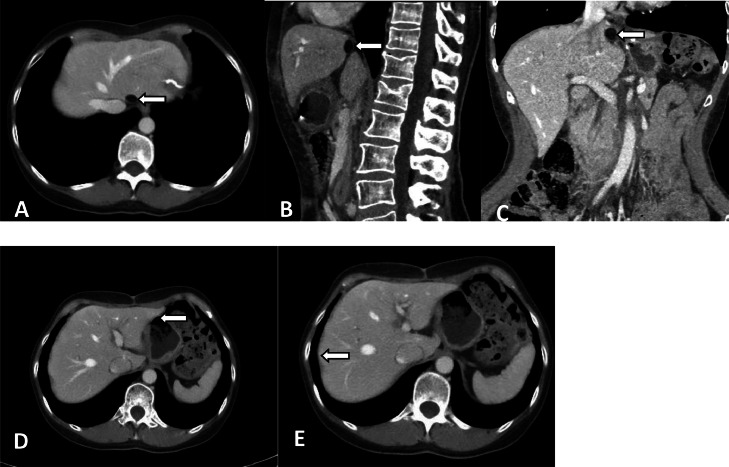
Reexamination using computed tomography (CT). On May 9, 2018, re-examination via CT showed a dense, fatty lesions (A-C) in the space between the left and caudal lobes of the liver under the hepatic capsule. The lesions in the left and right lobes of the original liver had disappeared (D, E).
